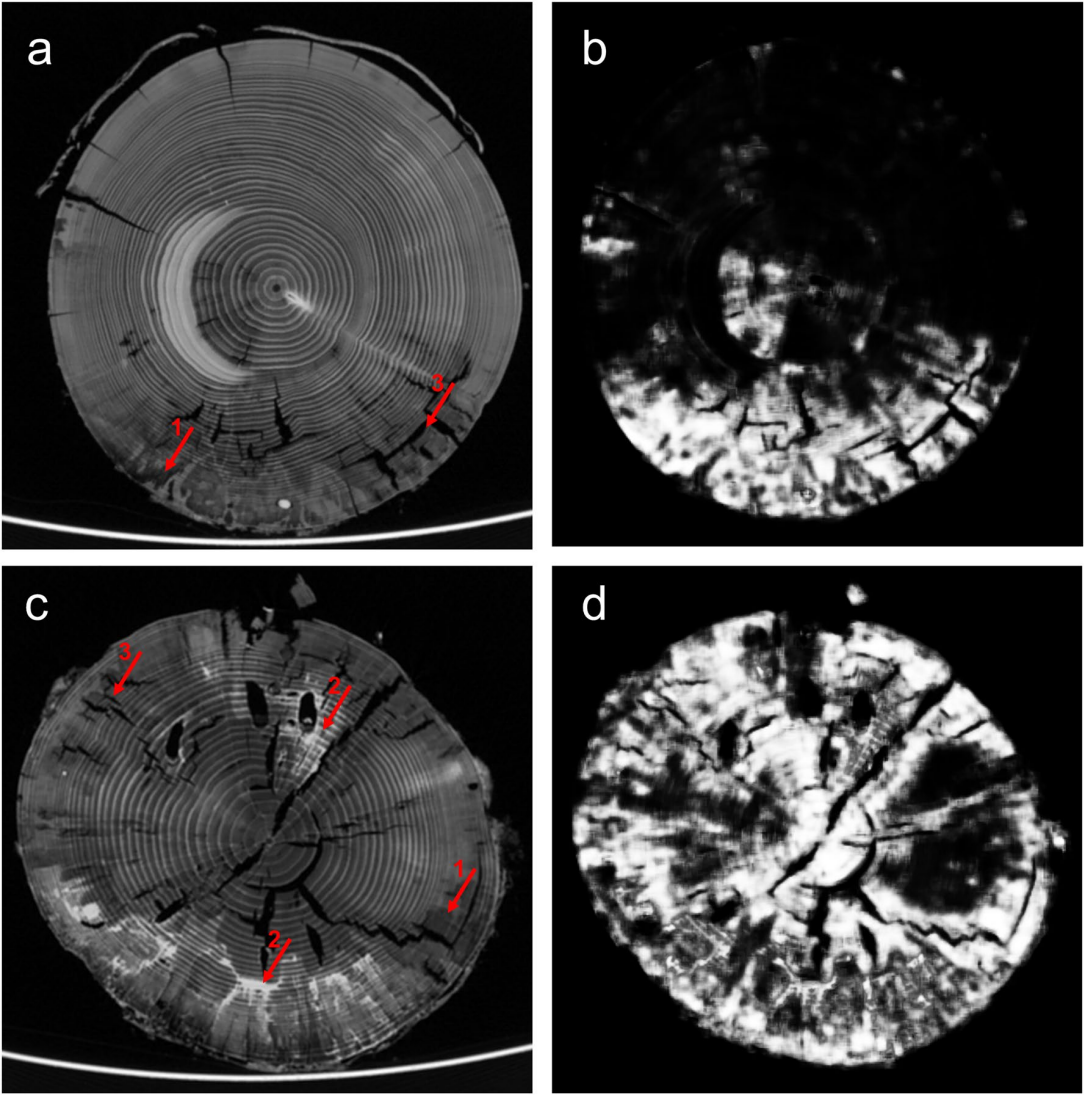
Computed tomography images of cross sections after 3.5 years of decomposition showing the raw images (a,c) and the corresponding prediction from the CNN model (b,d). White shading in b and d indicate a high probability of fungal decay, while dark shading indicate either wood with low probability of decay or cracks. Fungal decay was indicated either by darker (1) or brighter pixels (2) than the decayed wood or by perpendicular crack systems typical for brown rot (3). Note that cracks were not counted towards that area affected by fungal decay.
